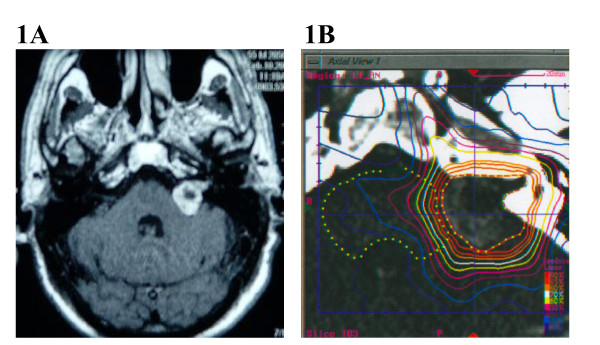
(A) A 51 year old woman presented with progressive hearing loss. An axial MRI of the brain After gadolinium administration demonstrated a left cerebellopontine angle acoustic neuroma. (B) Planning CT scan with IV contrast. The patient was treated with 2500 cGy to the 79% isodose line in five stages.
